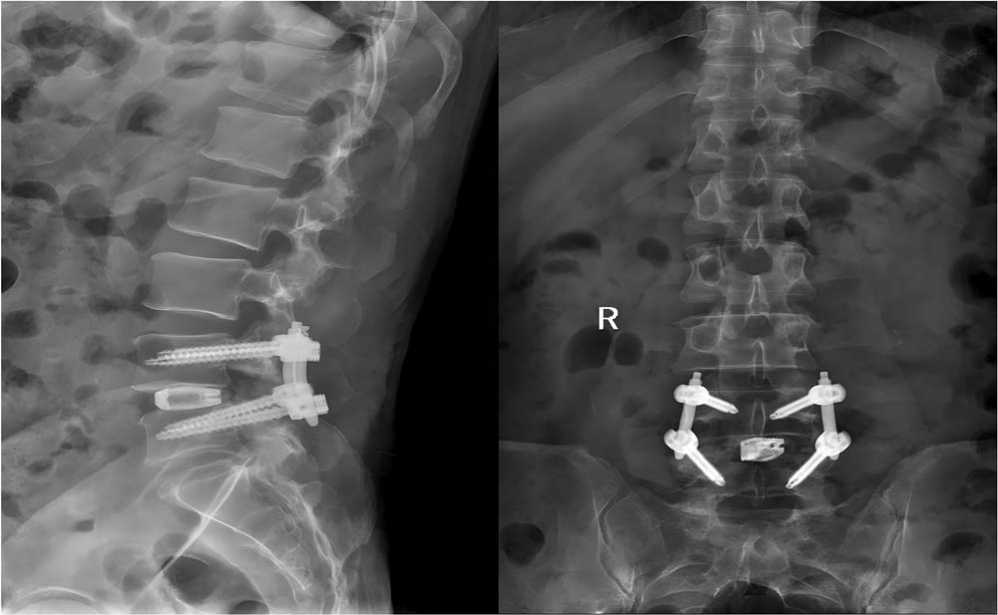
Postoperative anteroposterior and lateral radiographs of the lumbar spine.
